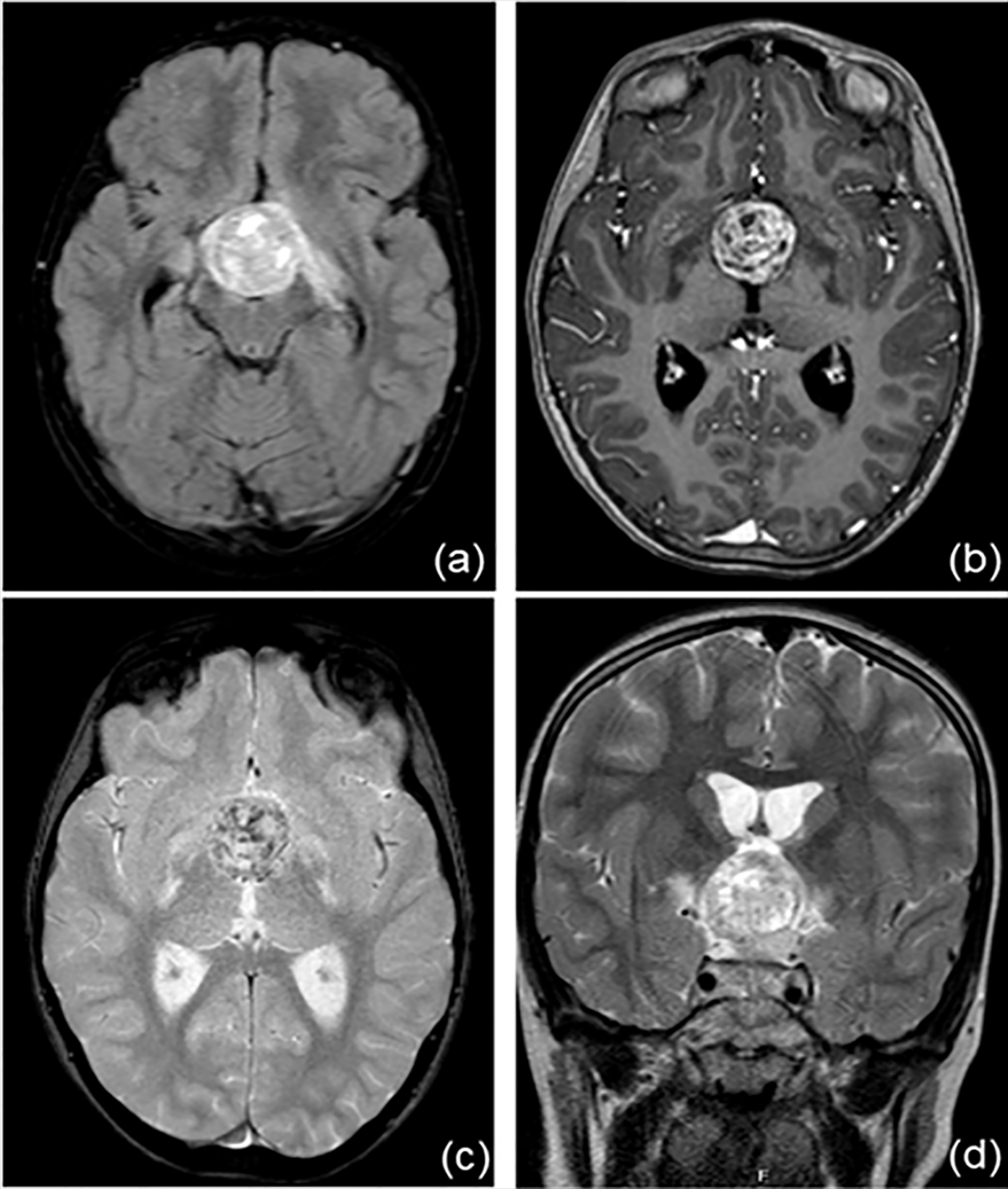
Brain magnetic resonance imaging at the time of diagnosis. There is a recognizable, voluminous expansive lesion at the hypothalamic level (A, FLAIR sequence) with an inhomogeneous structure, first read as calcifications (B, T1 sequence; C, T2 sequence). Optic chiasma, hypothalamic-pineal peduncle and mammillary body are not recognizable. (D, T2W_TSE sequence).
